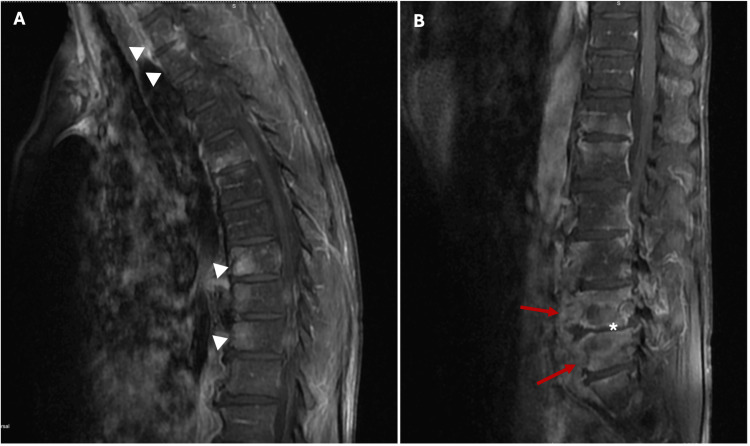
MRI of the dorsal and lumbar spine in a 57-year-old male with infectious spondylodiscitis A - Sagittal T1-weighted image of the dorsal spine; there is signal alteration with contrast enhancement after gadolinium injection in the D1, D2, D8, and D10 vertebrae (white arrowheads). B - Sagittal T1-weighted image of lumbar spine, L4 and L5, showing involvement of the somatic regions (red arrows), collapse of the disc space (asterisks), and a paravertebral tissue mass extending from L2 to S1. MRI - Magnetic resonance imaging
